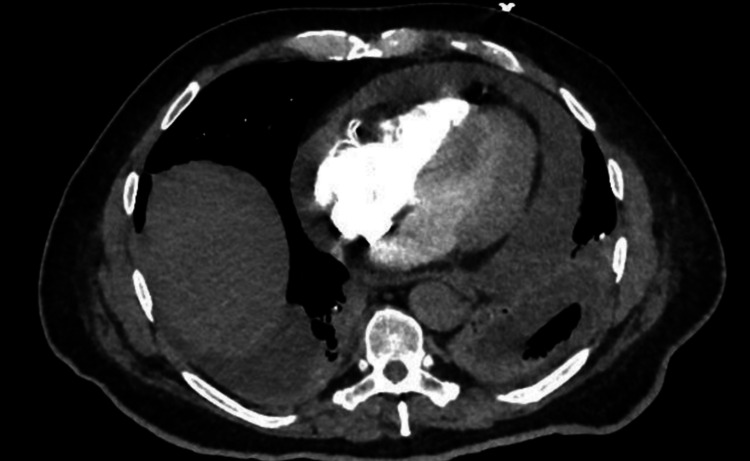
CT chest demonstrating large pericardial effusion CT: computed tomography
